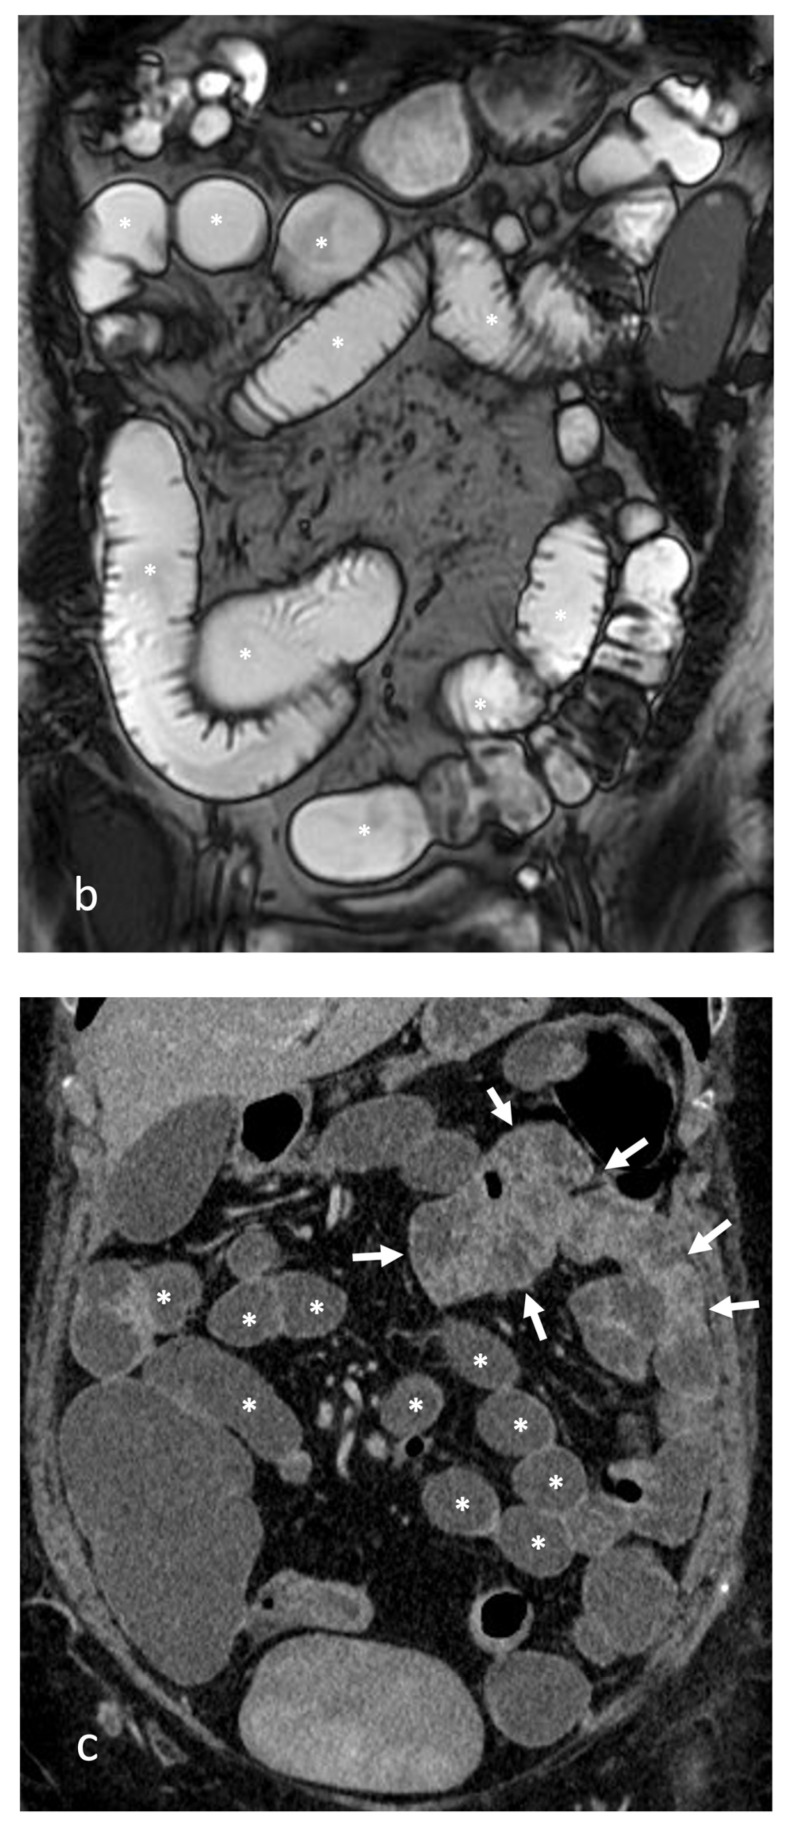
Small bowel distension in CT and MR enterography after oral administration of polyethylene glycol solution. (a) Coronal CT and (b) coronal T2-weighted MR images show great distention of the small bowel loops (asterisks), including both ileum and jejunum. (c) Coronal CT image of another patient shows good distention of the ileal loops (asterisks), whereas the jejunum is collapsed in the left hypochondrium (arrows).
